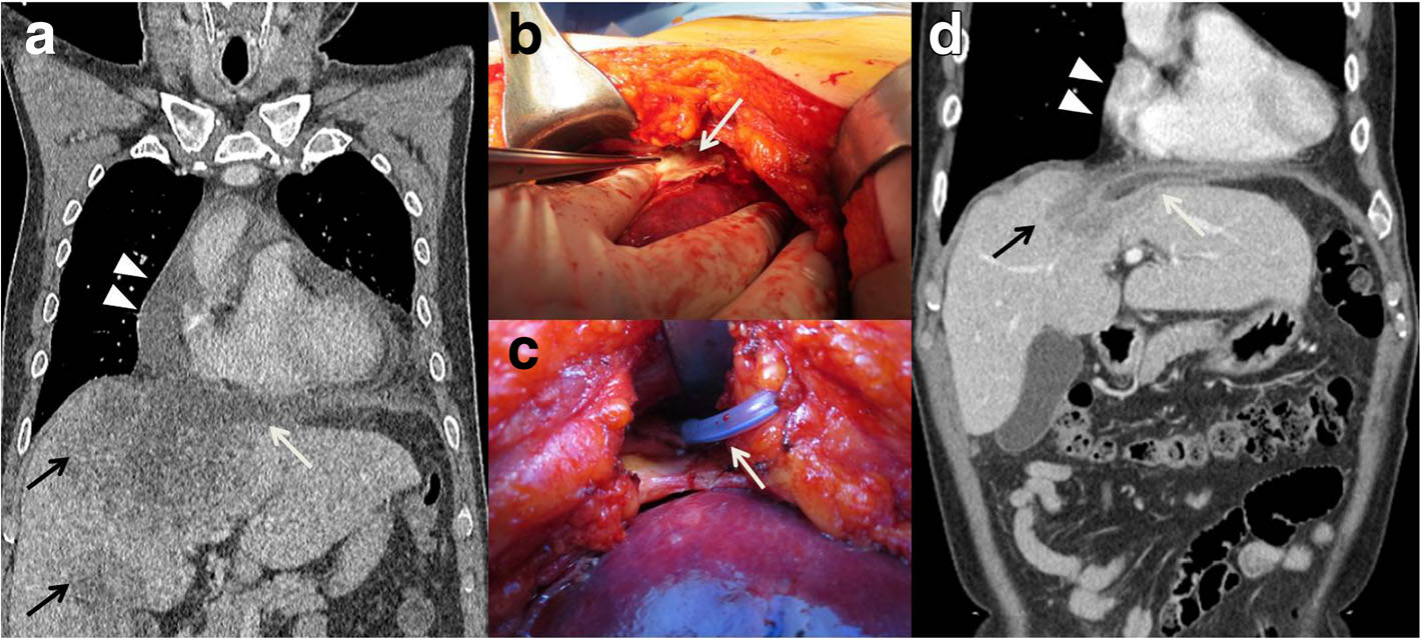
a. Computed tomography (CT) of chest demonstrated 10 × 6 × 8 cm (segment IV) and 4 × 4 × 4 cm (segment V) sized liver abscesses (black arrows), subphrenic fluid collection (white arrow), and moderate amount of pericaridal effusion (arrowheads). b. Intraoperative findings showed inflamed and necrotic diaphragm (white arrow) abutting liver abscess. c. After pericardial window was created, a pericardial drainage tube was inserted (white arrow). d. Follow-up abdomen CT after 20 days showed decreased size of liver abscess (black arrow) and subphremic fluid collection (white arrow), and complete resolution of pericardial effusion (arrowheads)
